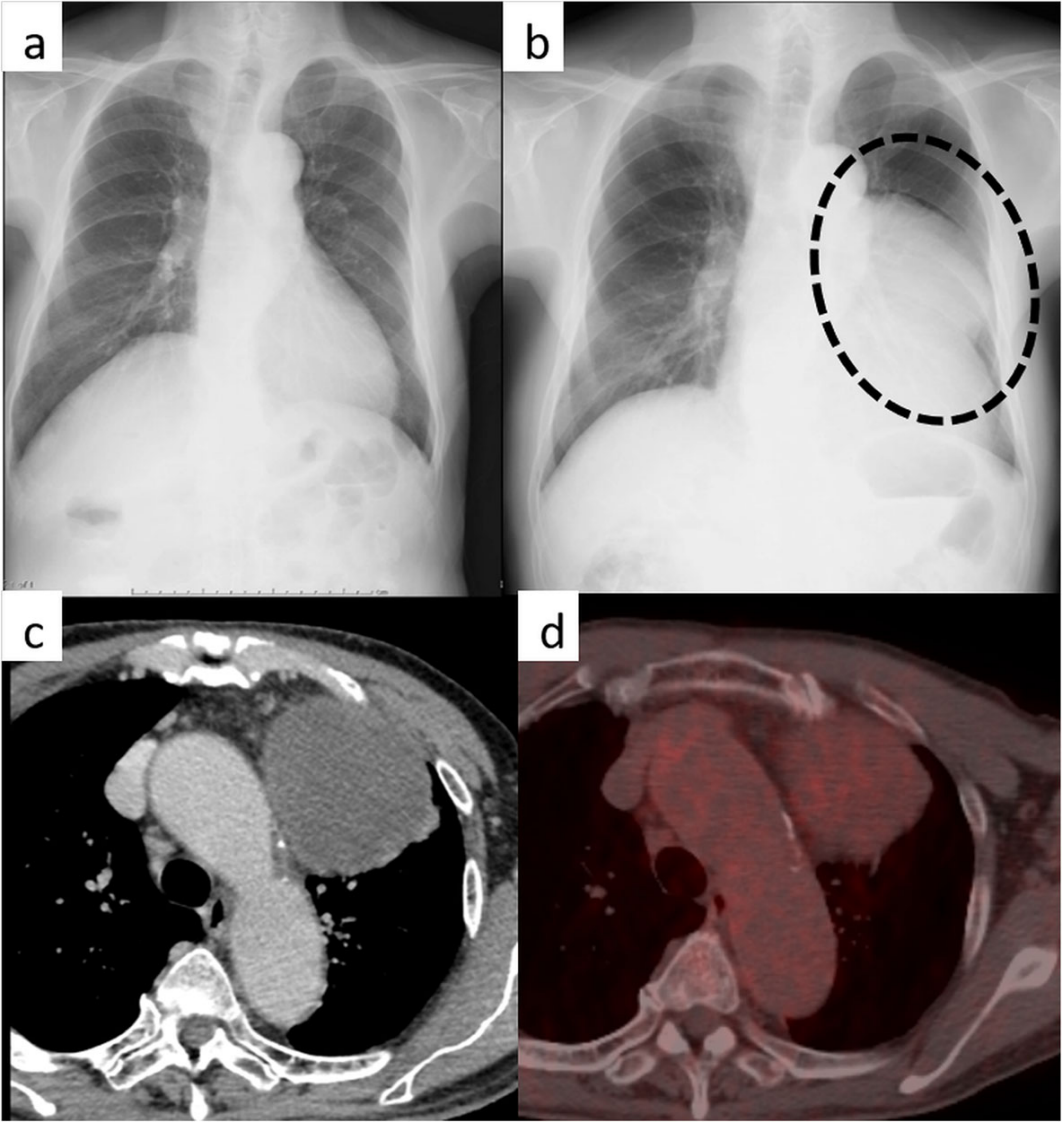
Chest radiography showing the mass (b: dotted circle). The mass was not observed a year ago (a). Chest-enhanced computed tomography showing an approximately 11-cm mediastinal tumor occupying left internal cavity (c). Fluorodeoxyglucose-positron emission tomography showing accumulation in the tumor (maximum standard uptake value = 3.3) (d)
